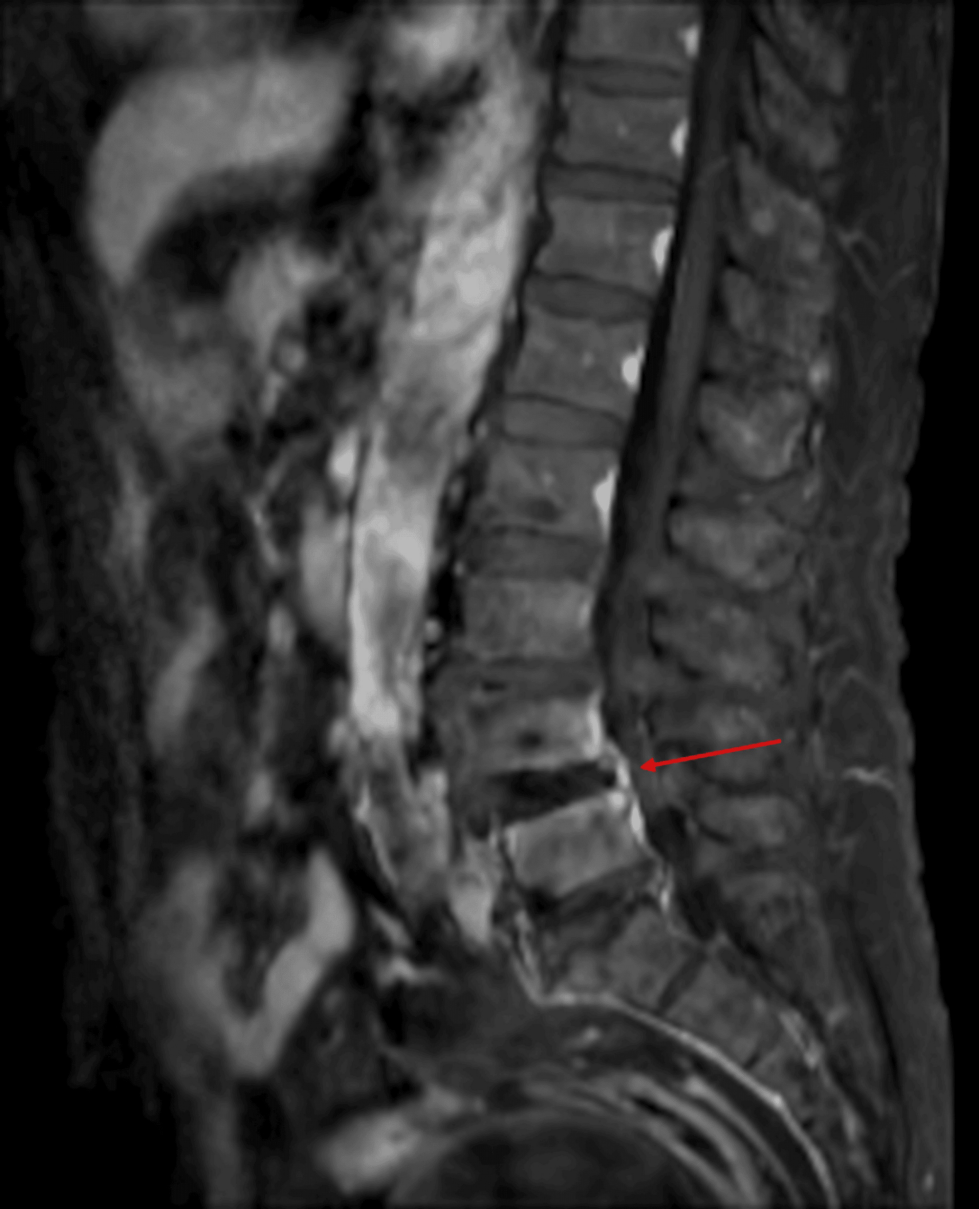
Follow-up contrast-enhanced lumbar MRI 19 days after the procedure. The red arrow indicates mildly thickened enhancing soft tissue in the anterior epidural space at L4/5. Contrast-enhanced lumbar MRI taken 19 days after the procedure. The red arrow highlights mildly thickened, enhancing soft tissue in the anterior epidural space at the L4/5 level, showing no significant interval change from the previous scan.
